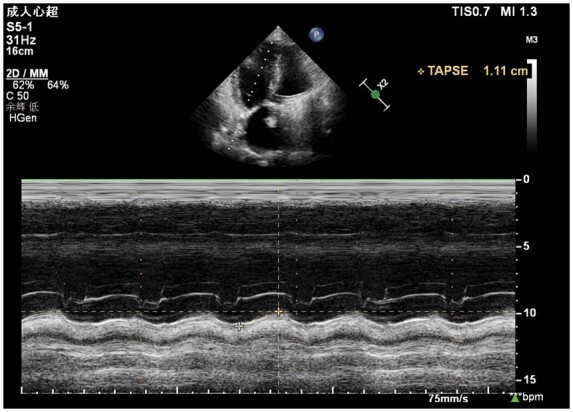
Transthoracic echocardiography showing right ventricular dysfunction.
